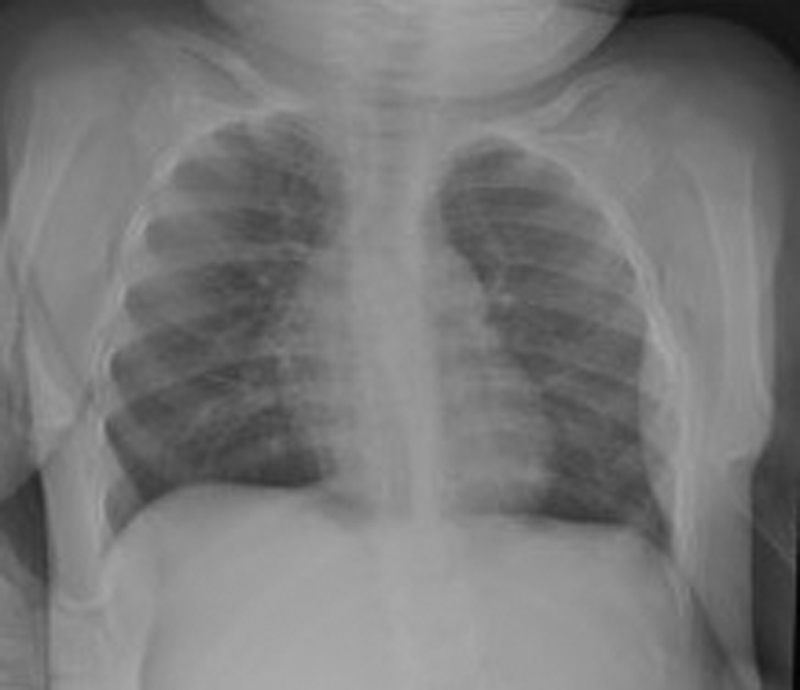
Chest X-ray at 1 year postoperative.
